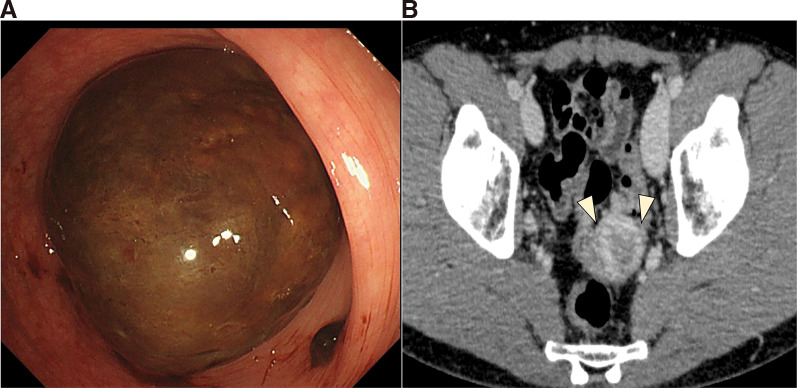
Preoperative findings. (A) Lower gastrointestinal endoscopy findings. A type 1 tumor, measuring 40 mm was observed in the sigmoid colon. (B) Contrast-enhanced CT findings. A tumor with enhancement, measuring 40 mm, was observed in the sigmoid colon, but there was no clear evidence of a lymph node or distant metastasis.
